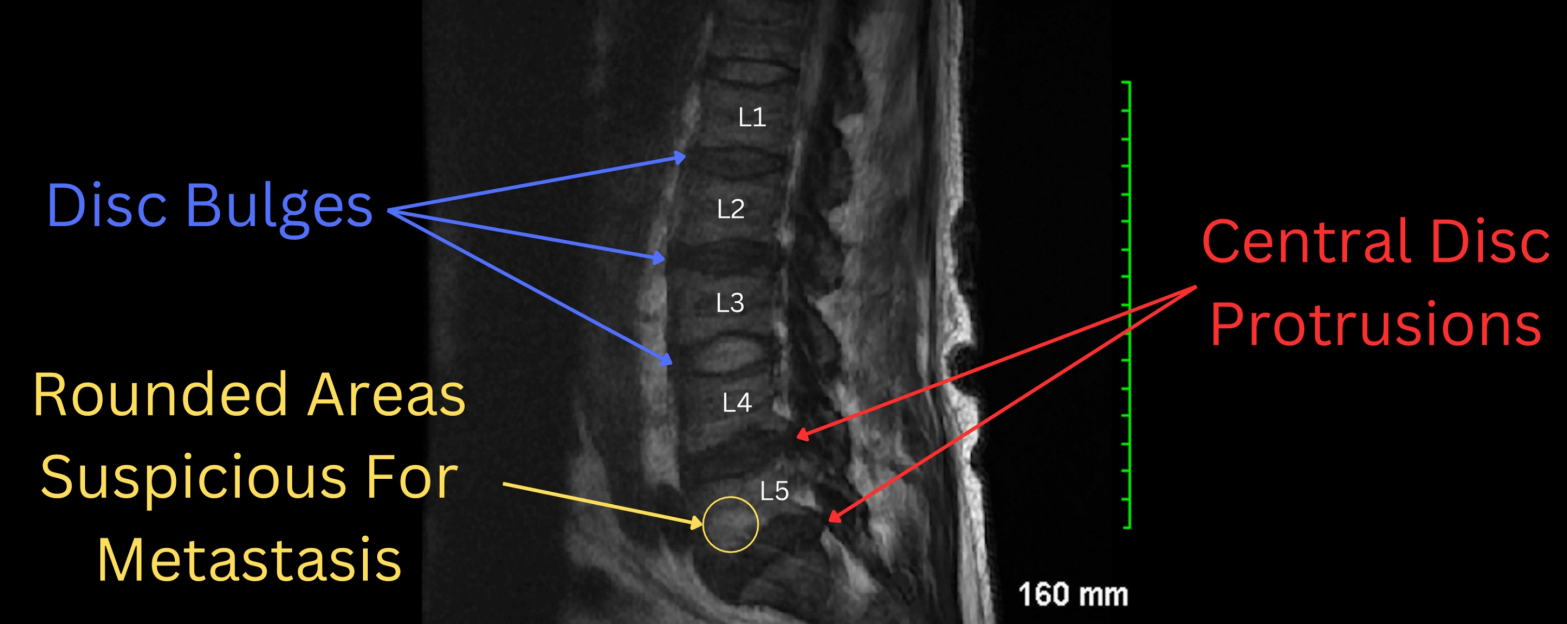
The MRI scan reveals the following findings at various lumbar levels. At L1-L2, there is a broad-based disc bulge (blue arrow) and mild facet changes. At L2-L3 and L3-L4, there are broad-based disc bulges (blue arrows) with moderate facet changes. At L4-L5, a large central disc protrusion (red arrow) is noted, accompanied by facet changes, and there is narrowing of the spinal canal. At L5-S1, a central disc protrusion (red arrow) with facet changes is observed. Additionally, the marrow signal is diffusely abnormal, with multiple rounded areas of abnormal signal (yellow circle and yellow arrow) that raise suspicion for possible metastatic disease. CT: computed tomography.
